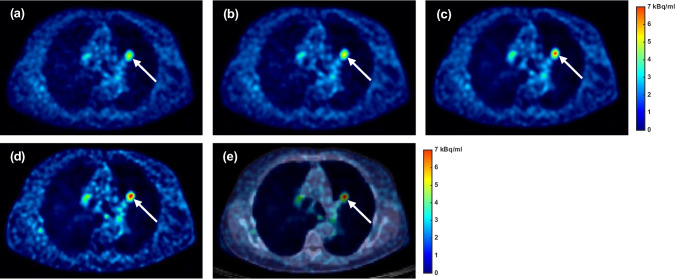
Image comparison for lung lesion. (a) Non-PRC. (b) Tissue-independent PRC. (c) TDSV PRC with 6.0 mm FWHM filter. (d) TDSV PRC with 4.0 mm FWHM filter. (e) PET/CT fusion for the TDSV PRC with 4.0 mm FWHM filter
